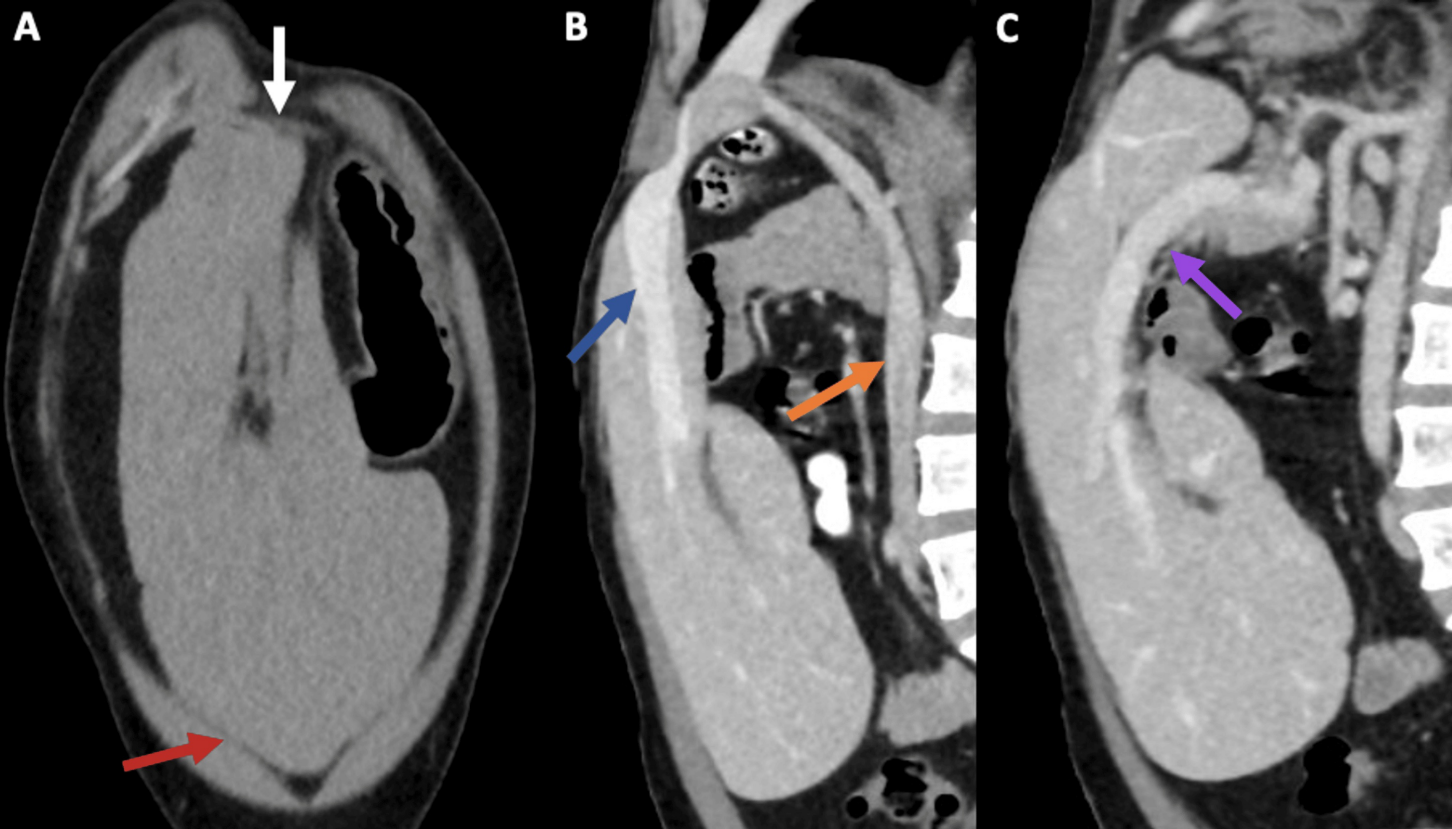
An abdominal CT scan (A) Coronal non-contrast CT scan showing a vertically orientated liver in the midline, with the right hepatic lobe located caudally (red arrow); notice that the left hepatic triangular ligament is anchoring the left hepatic lobe to the diaphragm (white arrow). (B)-(C) Sagittal contrast-enhanced CT scan in venous phase showing that the hepatic hilum is located cephalically; notice the suprahepatic veins (blue arrow), inferior vena cava (orange arrow), and the portal vein (purple arrow). CT: Computed tomography
